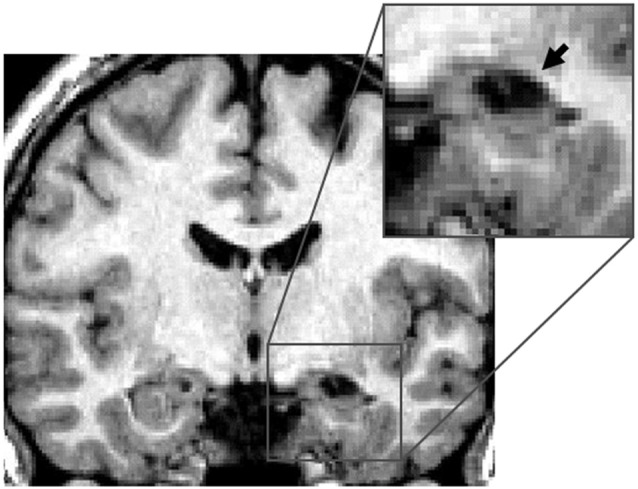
Right hippocampal lesion. MRI section in a coronal plane, zooming-in on the lesion to the right hippocampus (RH) of patient KJ (indicated with the arrow). This section was taken 12 mm posterior to the anterior commissure (*Y* = −12) in Talairach standard stereotaxic space (Talairach and Tournoux, 1988).
